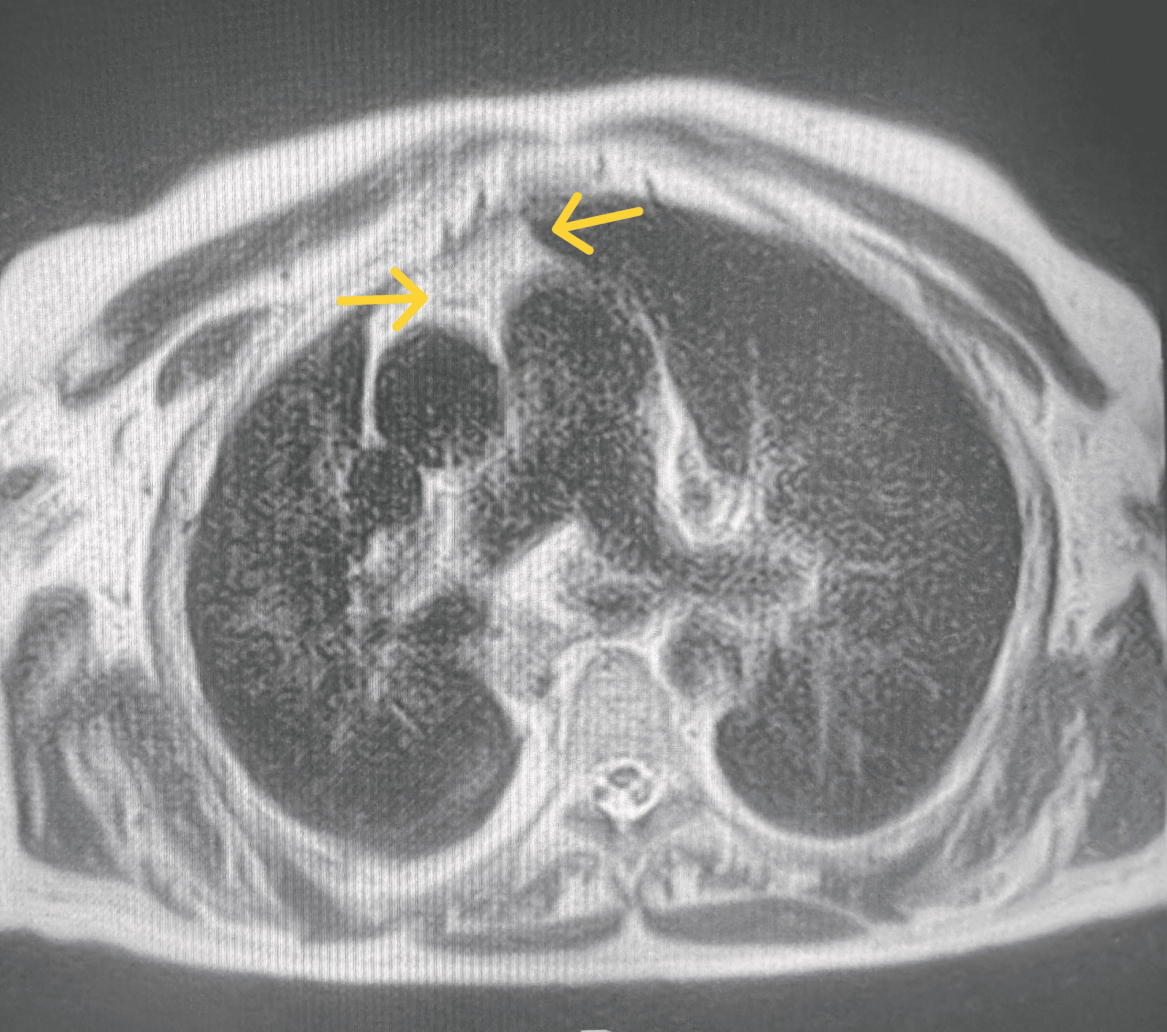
Chest MRI image of the patient MRI: magnetic resonance imaging
